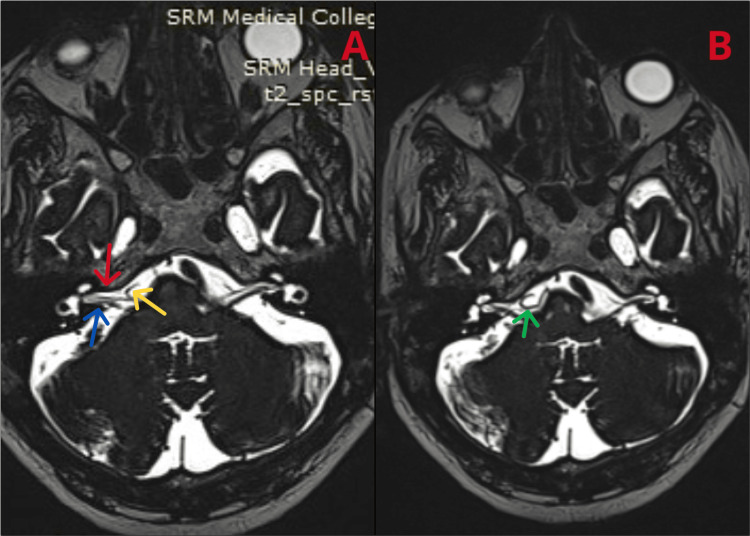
MRI of the brain revealing a (A) vascular loop (yellow arrow) of the AICA abutting the seventh (red arrow) and eighth (blue arrow) cranial nerve complexes on the right side in the CP angle cistern and (B) the AICA loop (green arrow). MRI: magnetic resonance imaging; MRA: magnetic resonance angiography; MRV: magnetic resonance venography; AICA: anterior inferior cerebellar artery; CP: cerebellopontine
